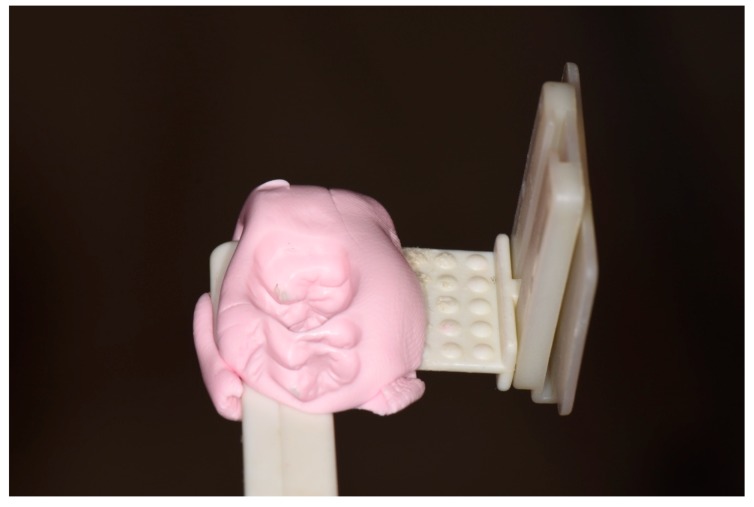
Custom bite blocks for periapical radiographs.
